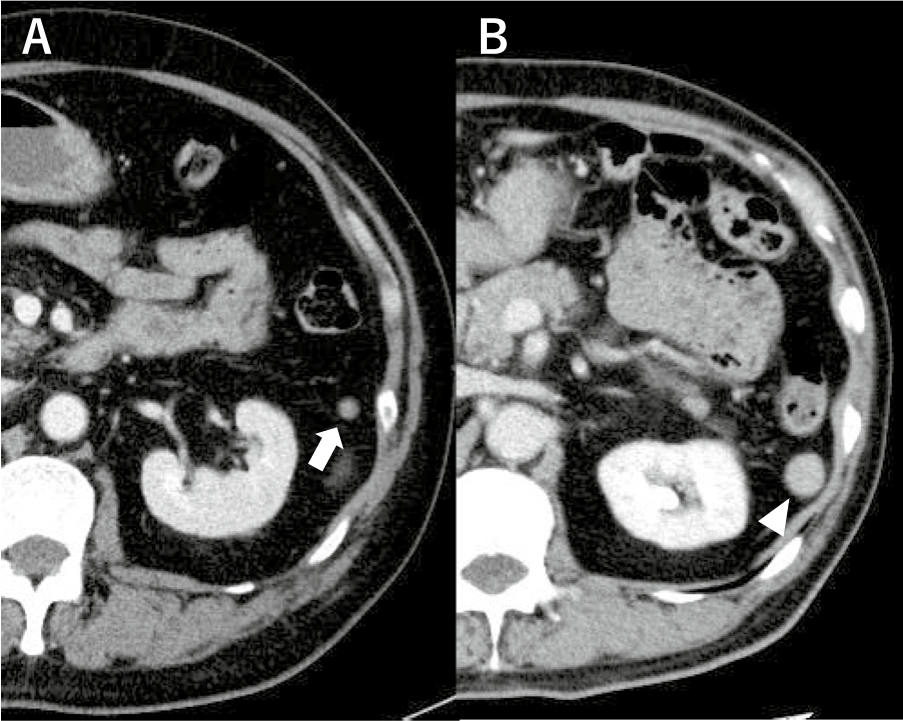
Contrast-enhanced CT scan depicting accessory spleen hypertrophy: prior to the initial LS, the accessory spleen measured 7 mm (arrow). It expanded to 16 mm upon AIHA recurrence (arrowhead). A pre-laparoscopic splenectomy; B at AIHA recurrence
